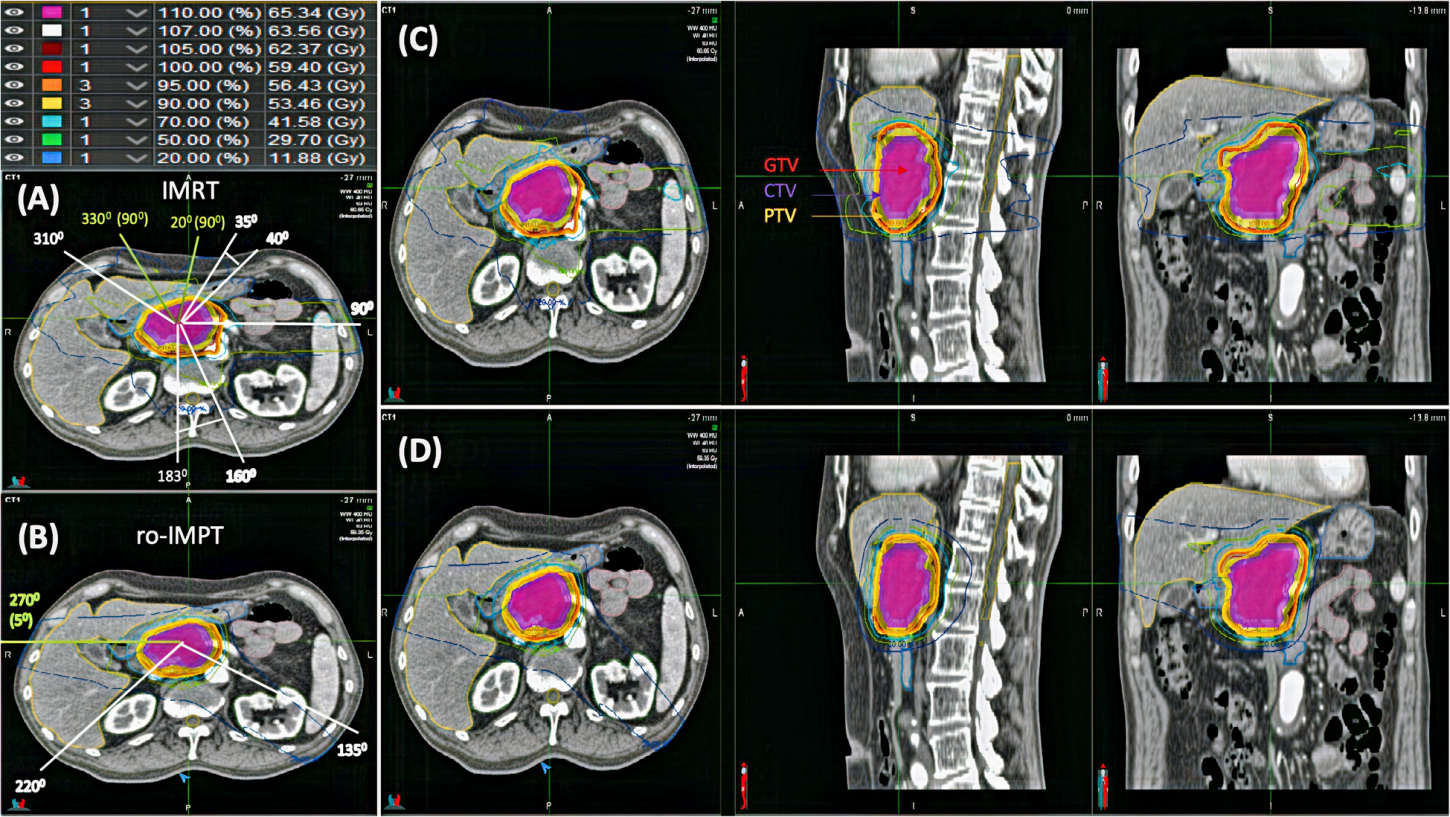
Overview of the beam configuration (a and b) and axial, sagittal, and coronal CT slices showing dose distribution of IMRT (c) and ro-IMPT (d) treatment plan. The range of beam direction and couch angle in all patients is given per beam direction. Non-coplanar beam direction is marked in green, and co-planar beam direction in white
